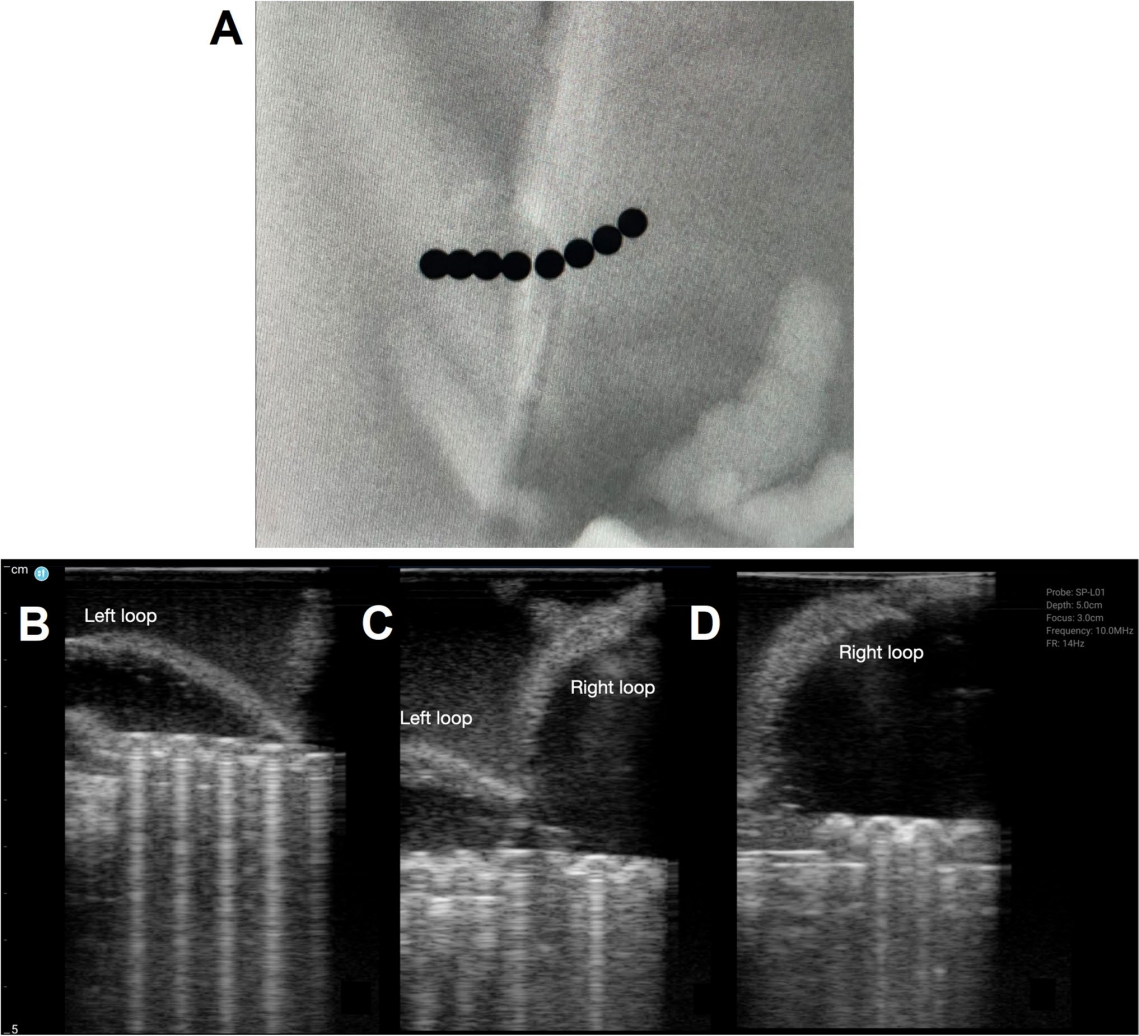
Eight Buckyball magnets in a linear orientation. A X-ray appearance of 8 Buckyball magnets. Four Buckyball magnets in two separate loops of bowel were tethered together by magnetic forces as seen on accompanying ultrasound images, with the (B) left loop, C both loops of bowel tethered by magnets with a “V” shape in between, and (D) right loop
